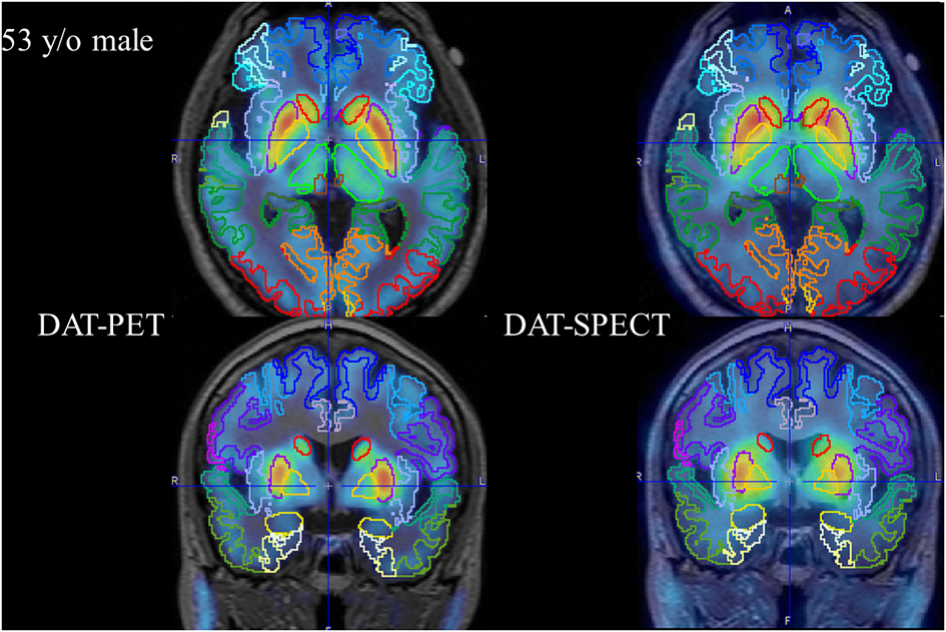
Representative case (53-year-old male). Axial and coronal fused PET (left column) and fused SPECT (right column) are shown.
